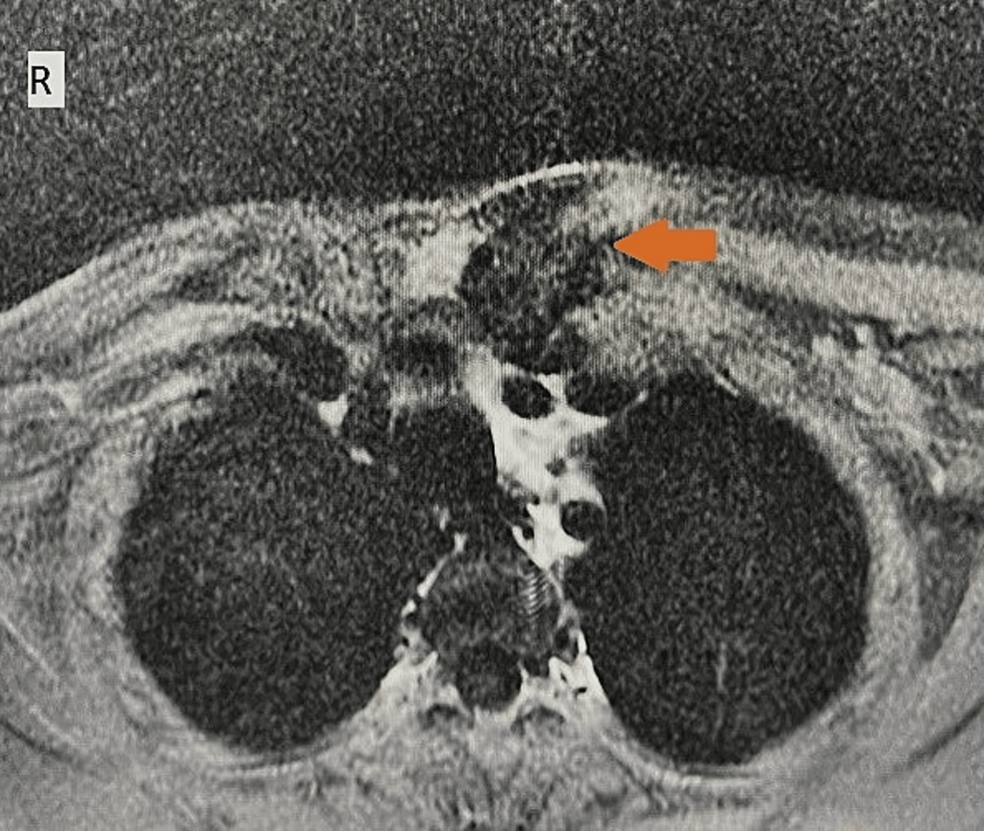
On the axial T1-weighted post-contrast image, the lesion shows heterogeneous enhancement (arrow).
